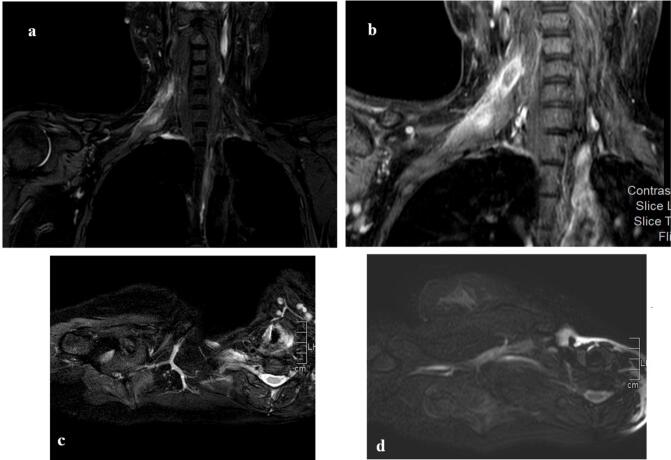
Fig. (1-a) T2 weighted brachial plexus MRI coronal cut. Fig. (1-b) T2 weighted brachial plexus MRI coronal cut. Fig. (1-c) T2 weighted brachial plexus MRI axial cut. Fig. (1-d) T2-weighted short-tau inversion recovery (STIR) brachial plexus MRI axial cut. Showing smooth thickening, hyperintense signal on fluid sensitive images and diffuse postcontrast enhancement involving C5 and to lesser extent C6 spinal roots, extending to the upper trunks, divisions, lateral and posterior cords of the right brachial plexus (red arrows). (For interpretation of the references to colour in this figure legend, the reader is referred to the web version of this article.)
